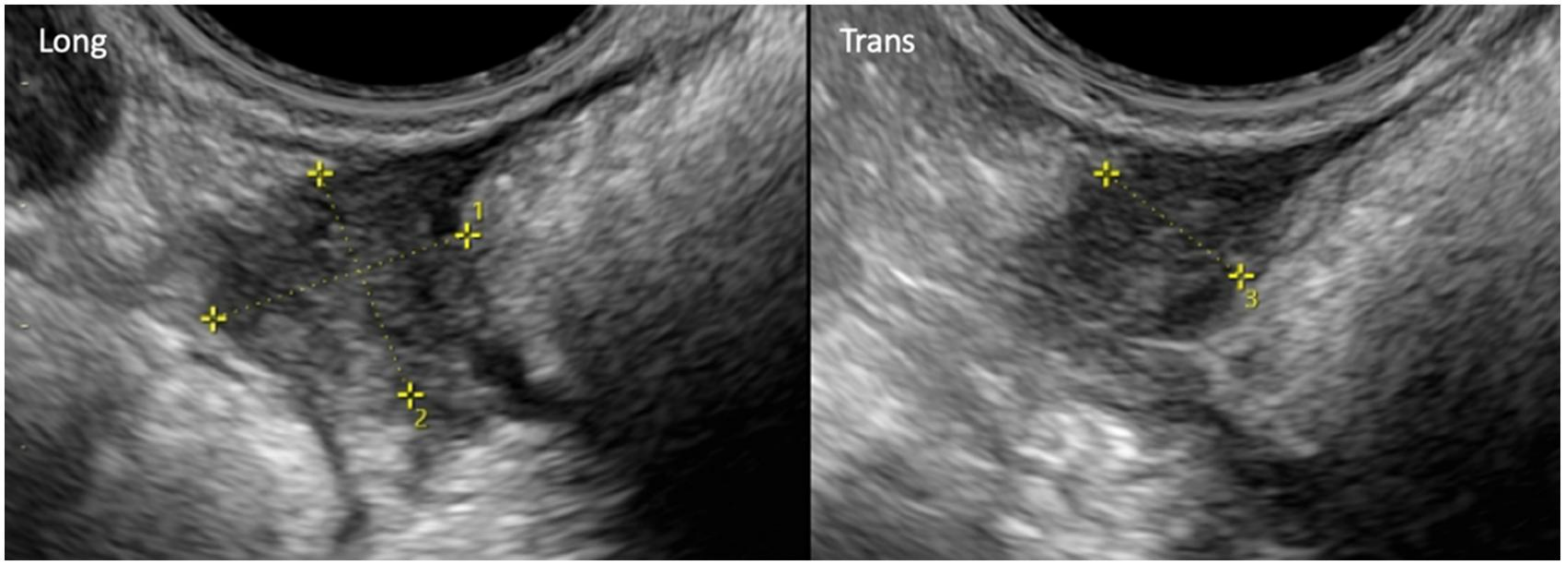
A 48-year-old female with a known small left ovarian endometrioma and a family history of ovarian and bowel cancer. Sonographic evaluation of the posterior compartment demonstrated a bowel nodule measuring 11 × 10 × 7 mm, likely involving the sigmoid colon, consistent with bowel deep endometriosis.
